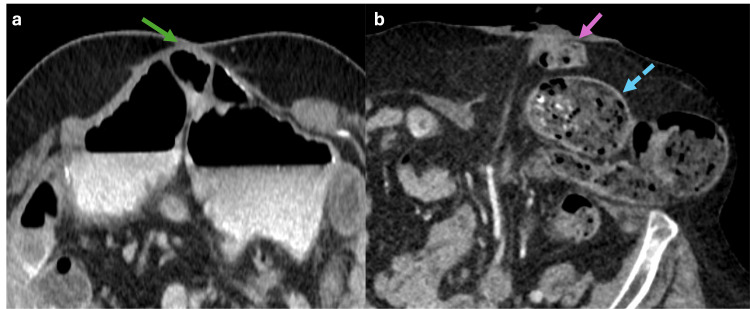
Axial abdominal CT scan with oral contrast showing an incisional hernia and parastomal hernia (a) Axial CT scan showing an anterior abdominal wall weakness with protrusion of transverse colon (green arrow) in a patient that underwent laparotomy; these findings consist of an incisional hernia. (b) Axial abdominal CT scan from another patient showing a colostomy stoma (pink arrow) with a parastomal protrusion of the descending colon (blue dotted arrow) consistent with a parastomal hernia with no signs of bowel obstruction. The figure was created by the authors.
